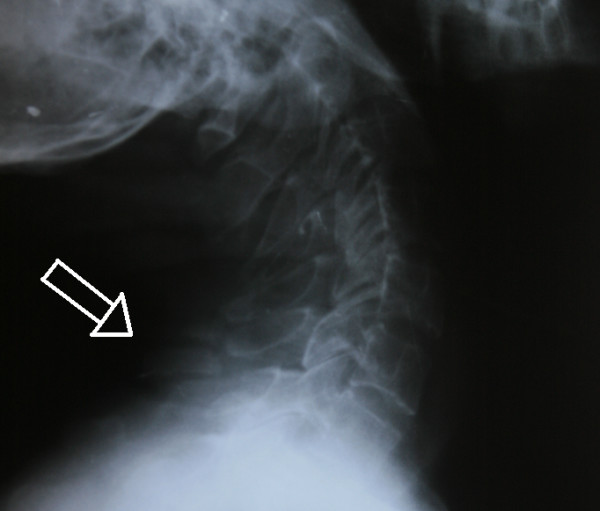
X-ray of the cervicodorsal site, lateral view. White arrow shows fractured spinous processes.
